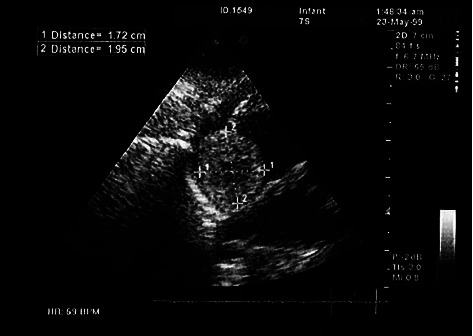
The echocardiography view of the lesion (a heterogeneous rounded atrial mass lesion (1.72 ✕ 1.95 cm^2^)).
